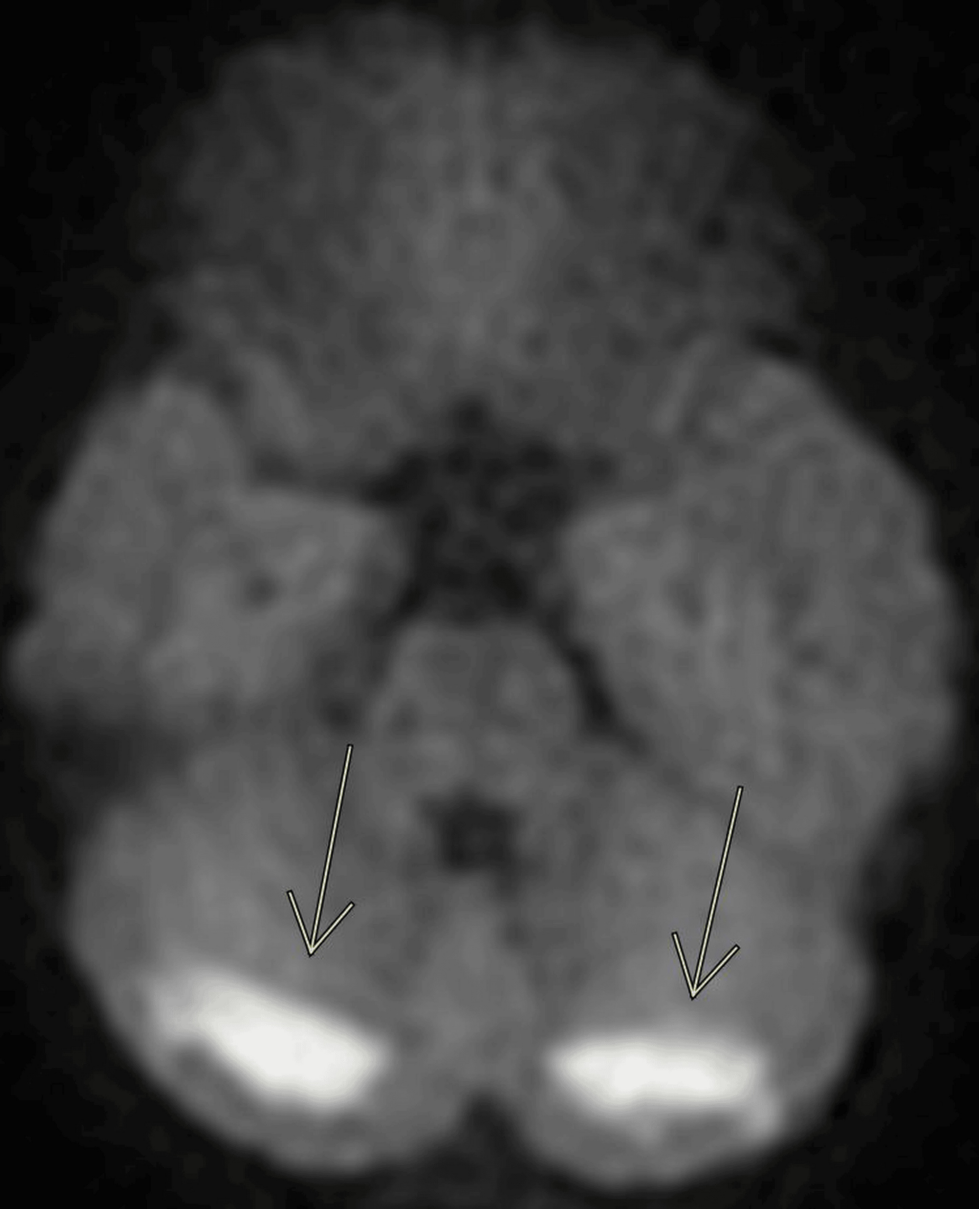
Brain MRI obtained on hospital day 3 Axial diffusion-weighted image (DWI) shows a bilateral hyperintense signal in the cerebellar white matter.
